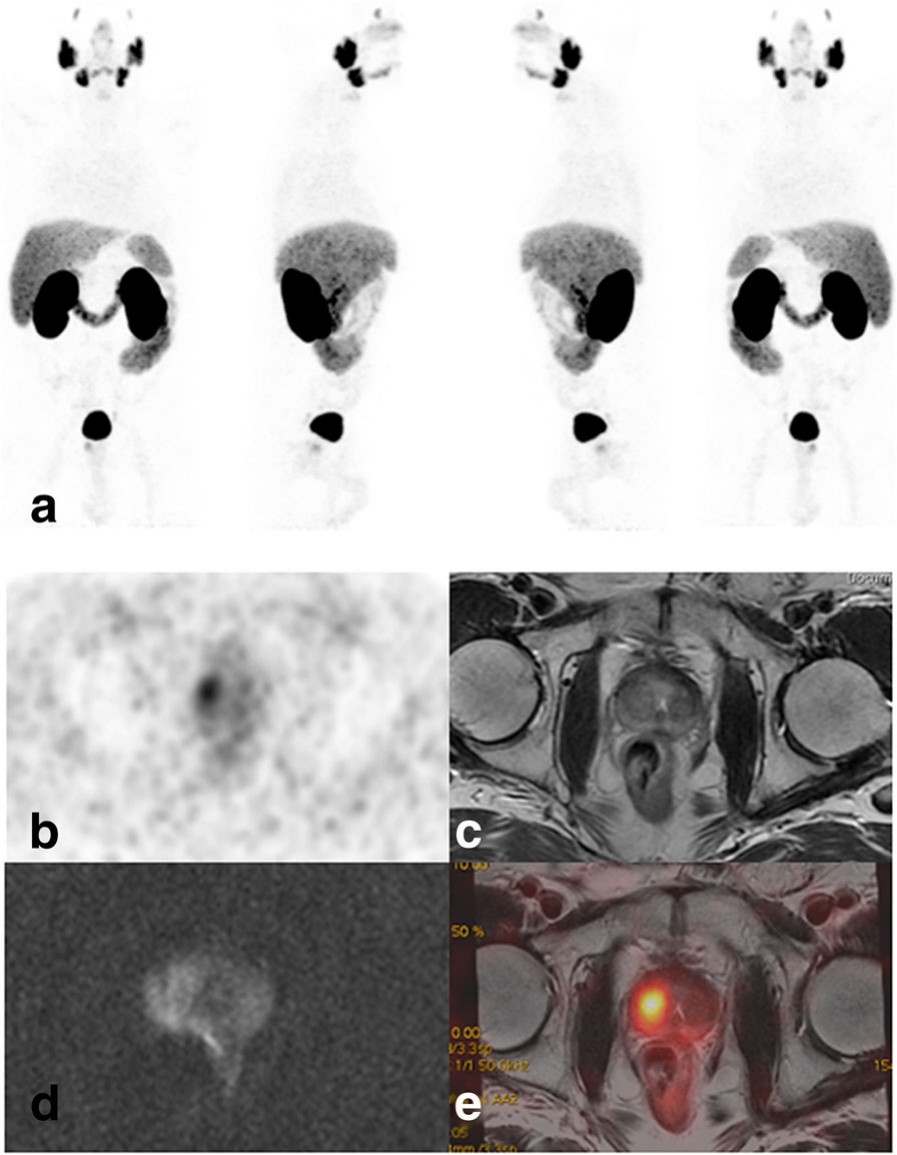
Patient 2. A 61-year-old-patient treated with radiotherapy and hormonotherapy with PSA relapse (PSA level 10 ng/ml). MIP and axial ^68^Ga-PSMA PET (a-b), high-resolution T2 (c), DWI (d) and PET/MR images (e) demonstrating a coincident local relapse. DWI shows peripheral diffusion restriction that partially overlaps the lesion seen in T2 and perfectly matches PSMA uptake
